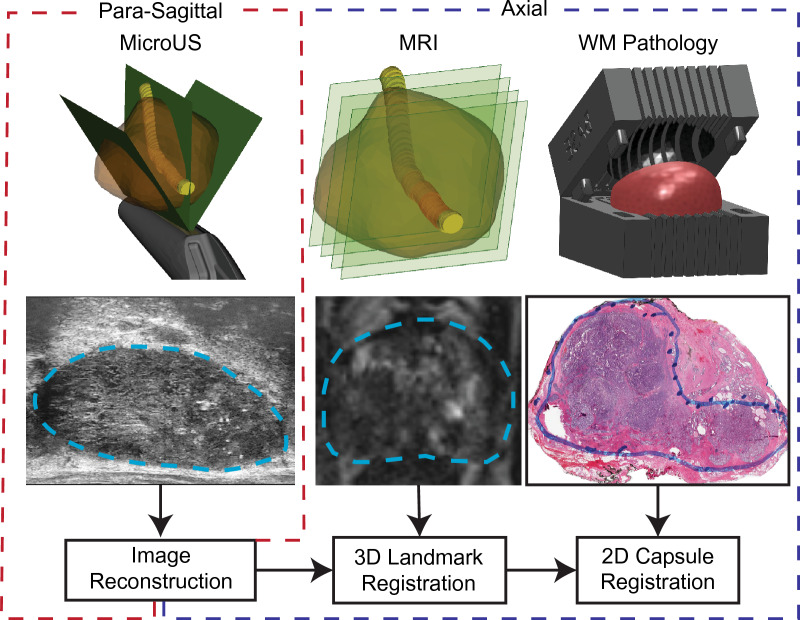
Overview of methodology of alignment of microUS images with MRI and WM pathology. MicroUS images are acquired in a para-sagittal fan sweep, while MRI is acquired in the axial plane. Following prostatectomy, the gland is sectioned in a pathology mold to align the WM sections with the MRI axial orientation. The para-sagittal microUS frames are reconstructed into a “true” sagittal orientation, then registered to MRI via a 3D landmark registration. Finally, MRI and microUS are registered to WM pathology via a 2D capsule registration.
